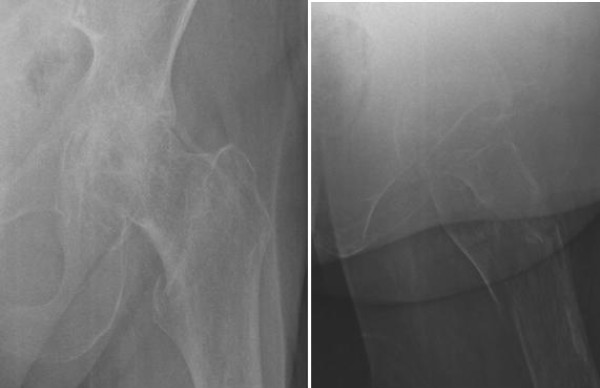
Case 2: preoperative radiographs of the left hip (anteroposterior and lateral views) demonstrating a comminuted intertrochanteric fracture, degenerative arthritis and osteopenia.
